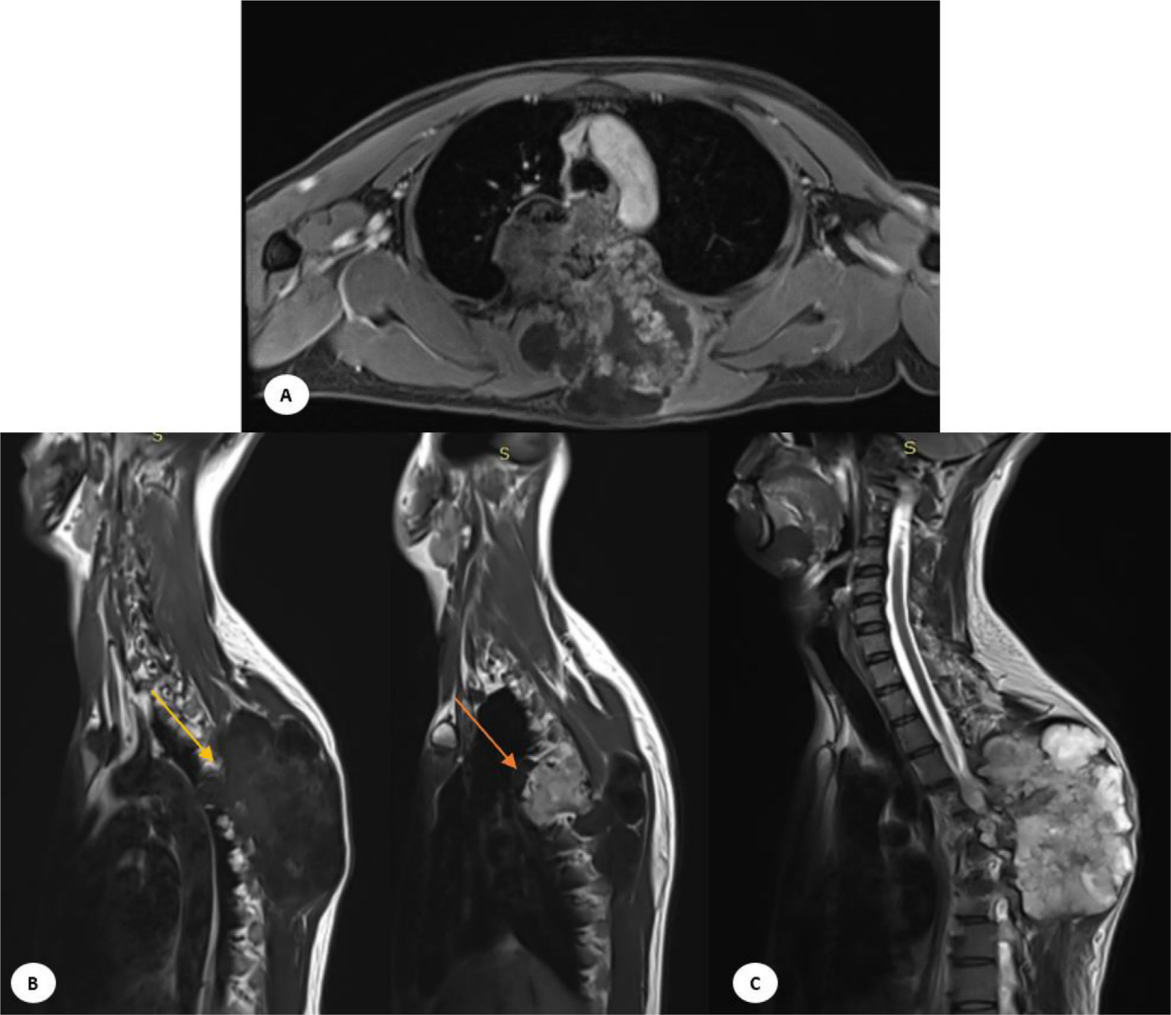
CT image in soft-tissue window (A, B) Axial and coronal view revealed in coronal and axial view the mass extending to the transverse process, costovertebral junction, and right posterolateral aspect of the thoracic vertebrae 5-6 (yellow arrow). (C, D) Axial and sagital view revealed an expansile sclerotic mass with a chondroid matrix located in the right posterolateral aspect of the thoracic vertebrae 5-6 (red arrow). MRI of the thoracolumbar region (MRI 1.5 T siemens Magnetom Essenza 2012). (A) T1-weighted imaging showed low signal intensity mass extending into the subcutaneous tissue in the bilateral posterior region of the vertebrae on axial image, (B) The mass infiltrated intradurally and intramedullary, reaching up to the level of vertebrae T4-T5, also stenosis of the spinal canal on sagittal image, on the left side, it directly bordered the aortic arch (yellow arrow) and the mass extended into the thoracic cavity (orange arrow), (C) T2-weighted showed high signal intensity partial mass with enhanced septations after intravenous injection of contrast media.
